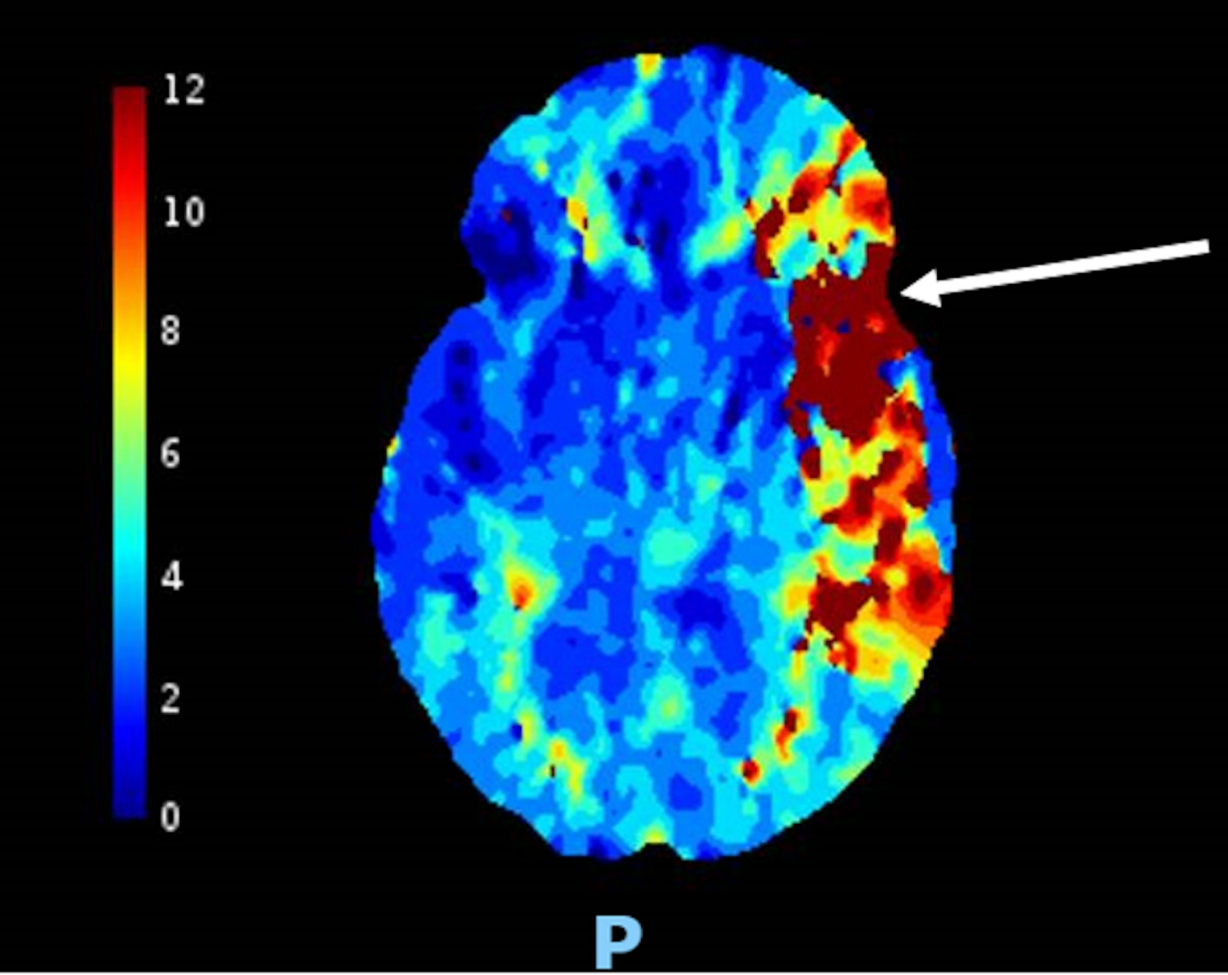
Computed tomography perfusion of the brain showing an acute core infarct with a large penumbra (red) in the inferior portion of the middle cerebral artery territory (white arrow).
